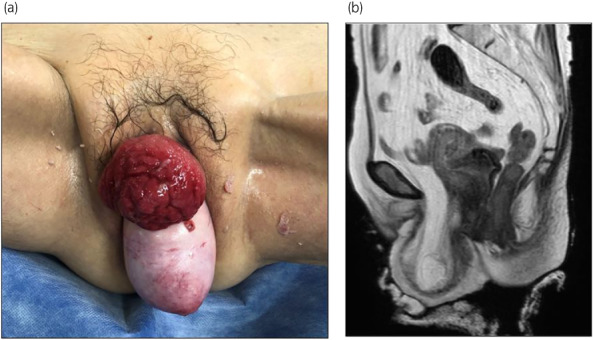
(a) Pelvic examination at the first visit. Legend: Uterine prolapse and protrusion of a red mass on its ventral side. (b) Magnetic resonance imaging at the first visit (T2‐weighted image). Legend: Bladder lumen could not be confirmed.
